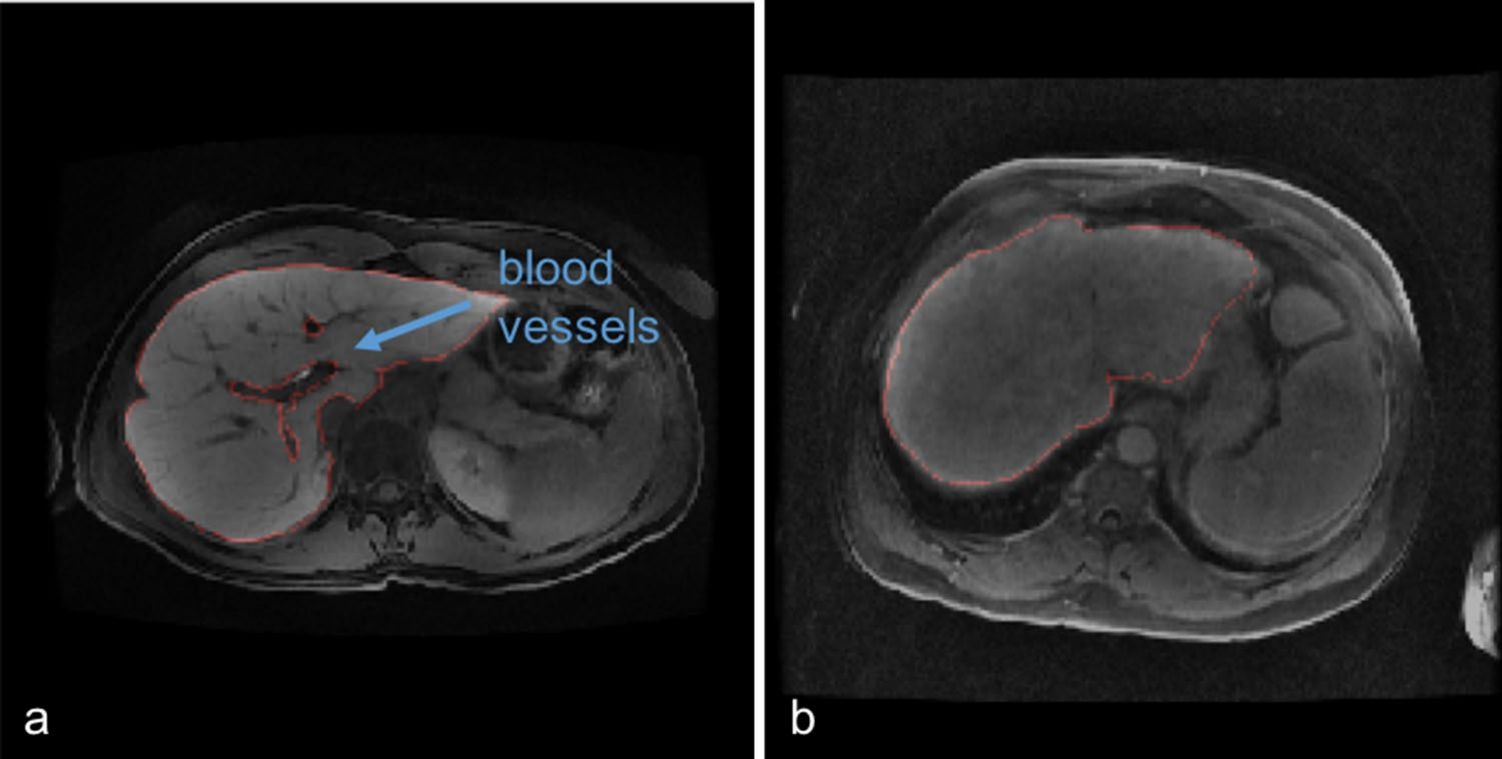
HBP MR images acquired at 20 min after the injection of hepatobiliary contrast agent and corresponding segmented liver regions (red line) (a) Adequate HBP: vessels are hypointense to background liver and clearly visible. (b) Suboptimal HBP: vessels are isointense to liver and difficult to identify.
